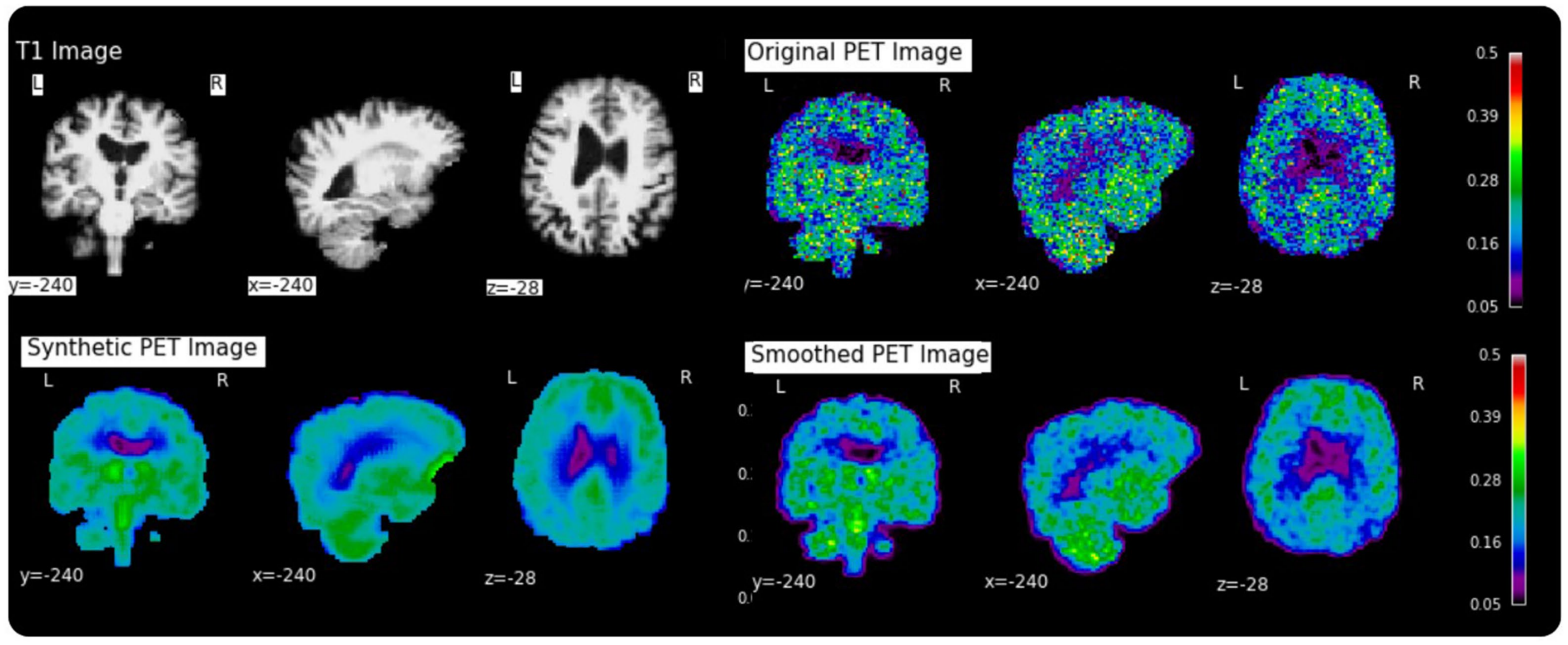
Examples of a random subject from the test set. The first row displays original intensity- normalized structural MRI and PET images, the second row presents our reconstructed images (left) and the original PET image post Gaussian smoothing (fwmh = 4 mm), (right).
